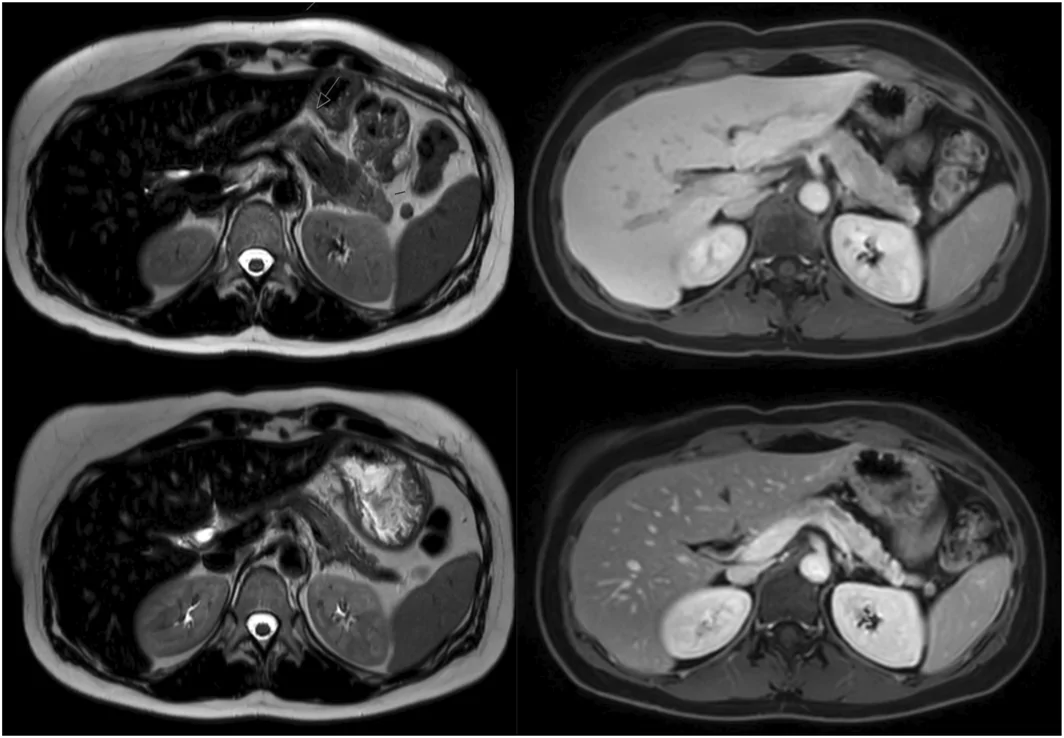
Radiological imaging of immune checkpoint inhibitor‐induced pancreatitis (patient 2). Magnetic resonance images (T2‐weight—left; diffusion‐weight—right) at the time of ICIPI diagnosis (03/2020, upper panel) and 2 months later following steroid therapy (lower panel). The typical image of an autoimmune pancreatitis with swelling of the gland, drawn contures, and vanishing of the main pancreatic duct are obvious as well as improvement after treatment.
